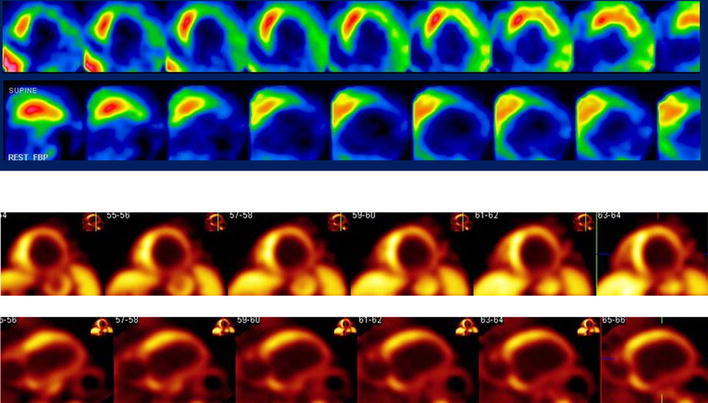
A series of short-axis slices of MIBG SPECT and HED PET images of a patient with heart failure. A moderate reduction of tracer uptake is noted, particularly in the inferior and posterolateral regions (Color figure online)
